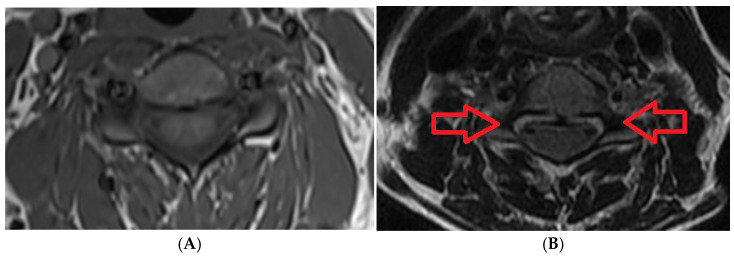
(A) Normal axial T1 MRI of cervical spine; (B) 57 year old female patient with C3–C4, C4–C5, C5–C6 and C6–C7 abnormalities: multiple levels of posterior disc osteophytic complexes indenting the ventral aspect of the subarachnoid space and encroaching upon neural foramina bilaterally at the corresponding levels.
